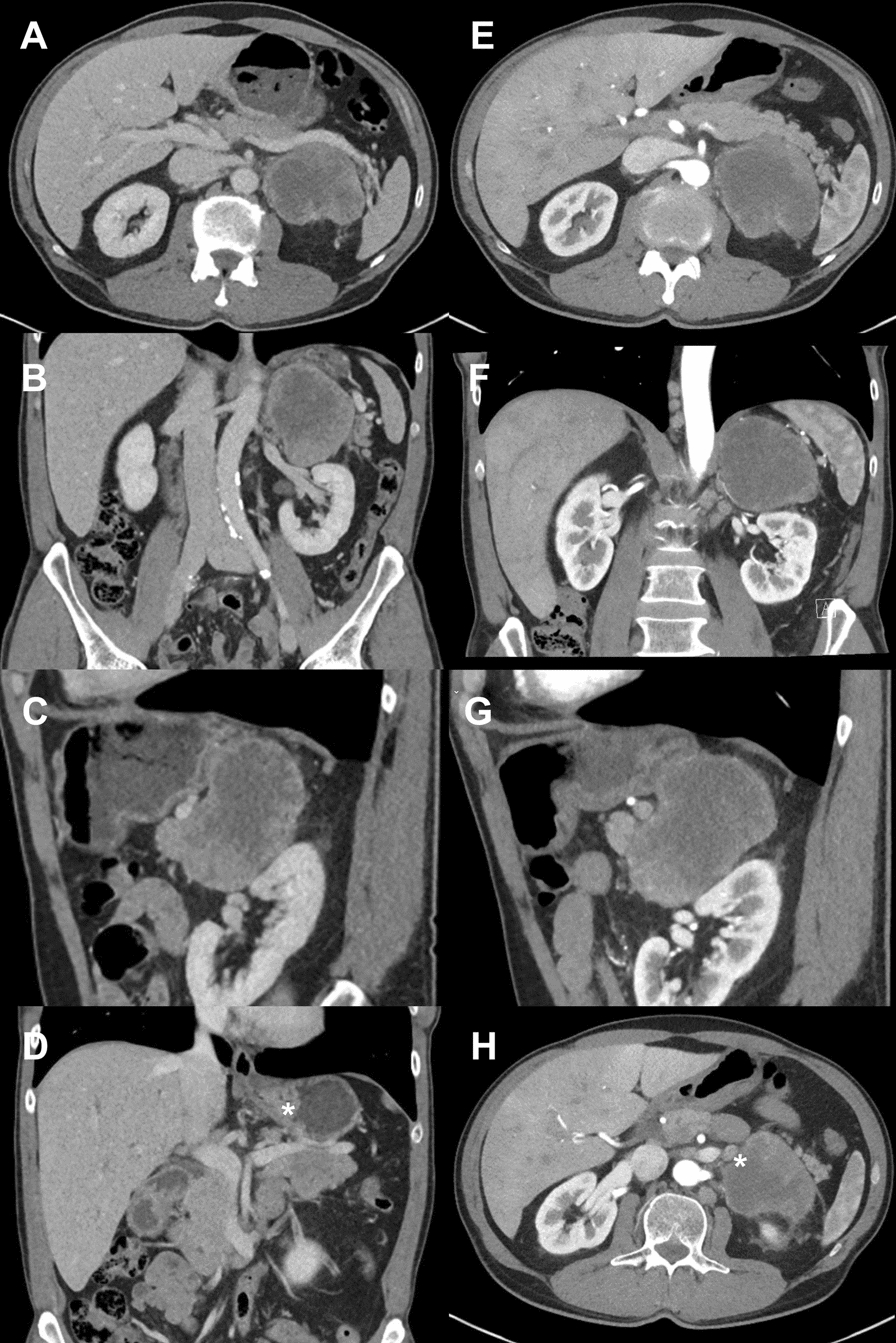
Computed tomography. A–D First computed tomography of the abdomen showing the large adrenal tumor on the left side; transverse (A), coronal (B), sagittal (C) section. ^*^Thickened gastric wall suspected to indicate infiltration of the adrenal tumor into the stomach (D). E–H Follow-up computed tomography a few weeks after the first computed tomography of the abdomen showing an increase in the large adrenal tumor on the left side; transverse (E), coronal (F), sagittal (G) section. ^*^Enlarged lymph node (14 mm) adjacent to the large adrenal tumor suspected to indicate a lymph node metastasis of the adrenal tumor (H)
